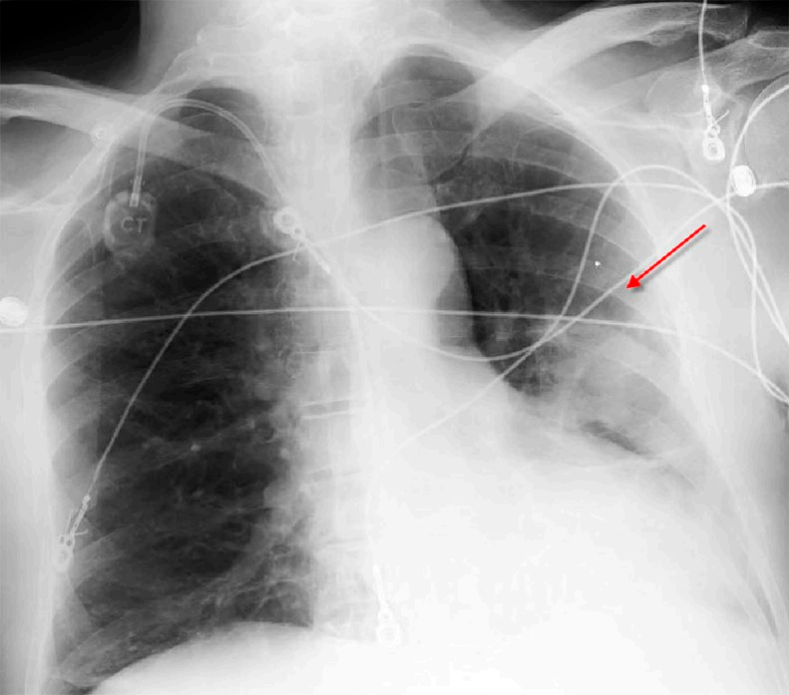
Posteroanterior view of the chest radiograph showing minimal pulmonary vascular congestion with left upper lobe opacity.
